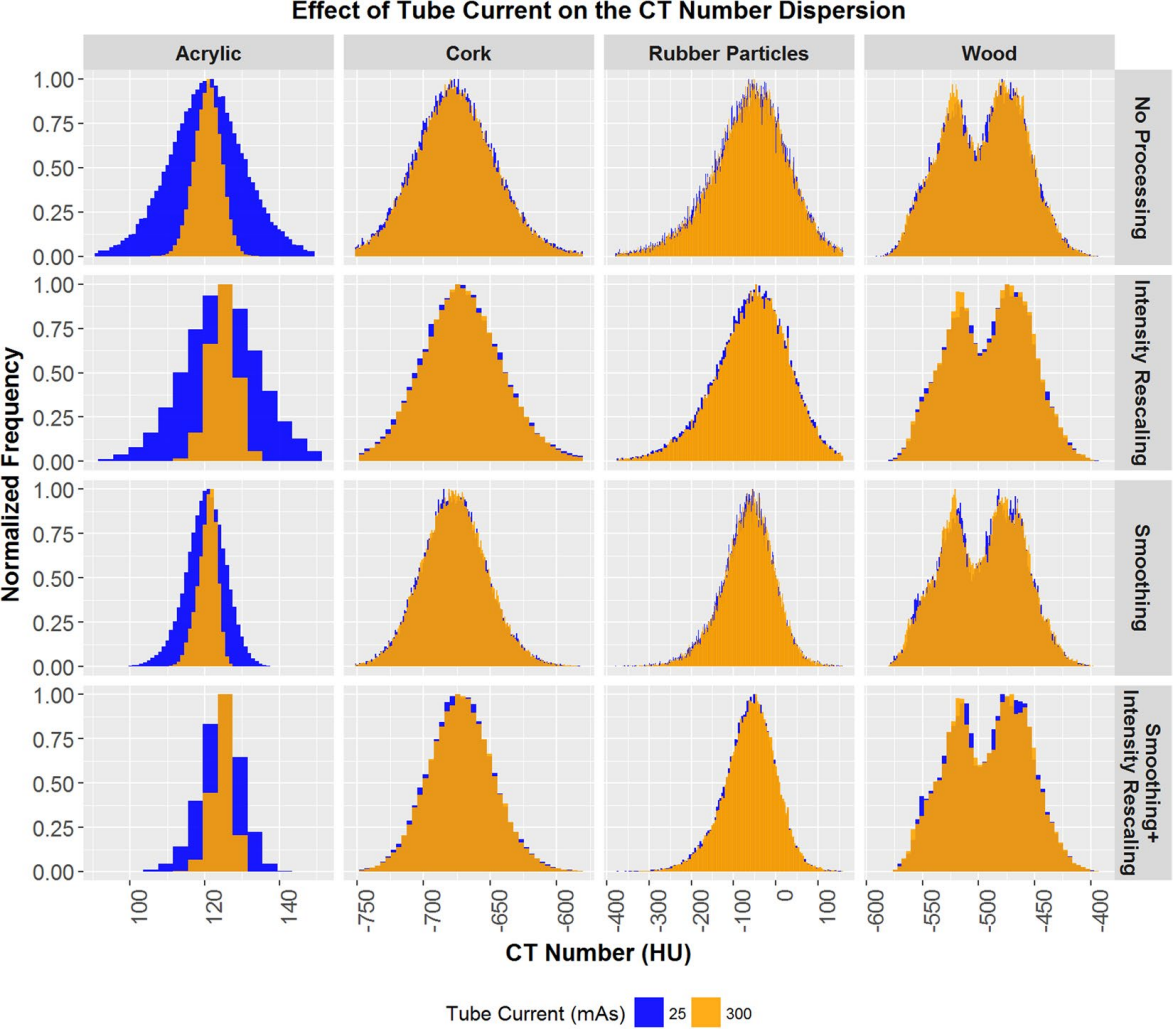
Image intensity histograms for the acrylic, cork, rubber particle, and sycamore wood cartridges acquired using 25 and 300 mA∙s tube current on a GE LightSpeed VCT computed tomography (CT) scanner. For display purposes, the frequency values have been rescaled so that the maximum is 1.0.
